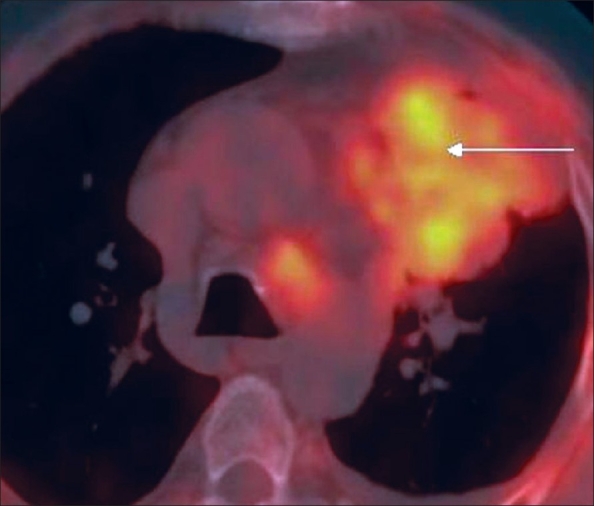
Fused PET/CT axial image gives morphological and physiological information, allowing proper planning of the FNAC site (arrow)
